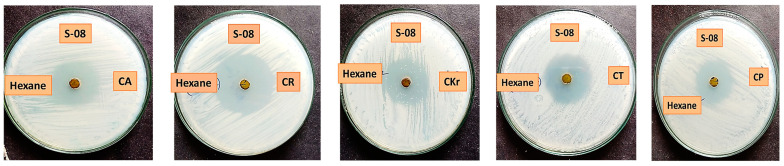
Anticandidal activity of saltmarsh plant *Launaea sarmentosa* extract exhibits the highest inhibition (CA—*Candida albicans*; CR—*Candida kefyr*; CKr—*Candida krusei;* CT—*Candida tropicalis*; CP—*Candida parapsilosis*).
